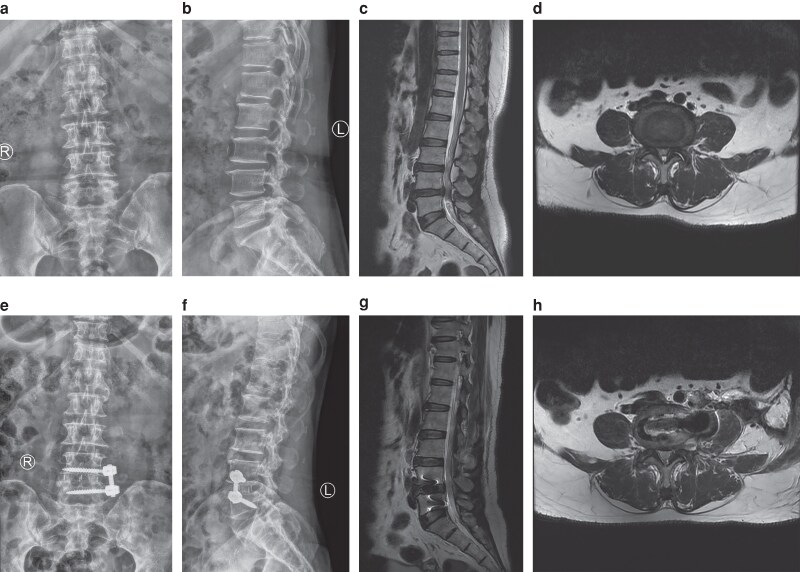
A 63-year-old female with lumbar disc herniation who underwent L4-5 oblique lumbar interbody fusion a, b preoperative anterolateral X-ray; c preoperative T2 sagittal MRI showed L4-5 herniation; d preoperative T2 axial MRI; e, f postoperative anterolateral X-ray; g preoperative T2 sagittal MRI; h preoperative T2 axial MRI. MRI, magnetic resonance imaging.
